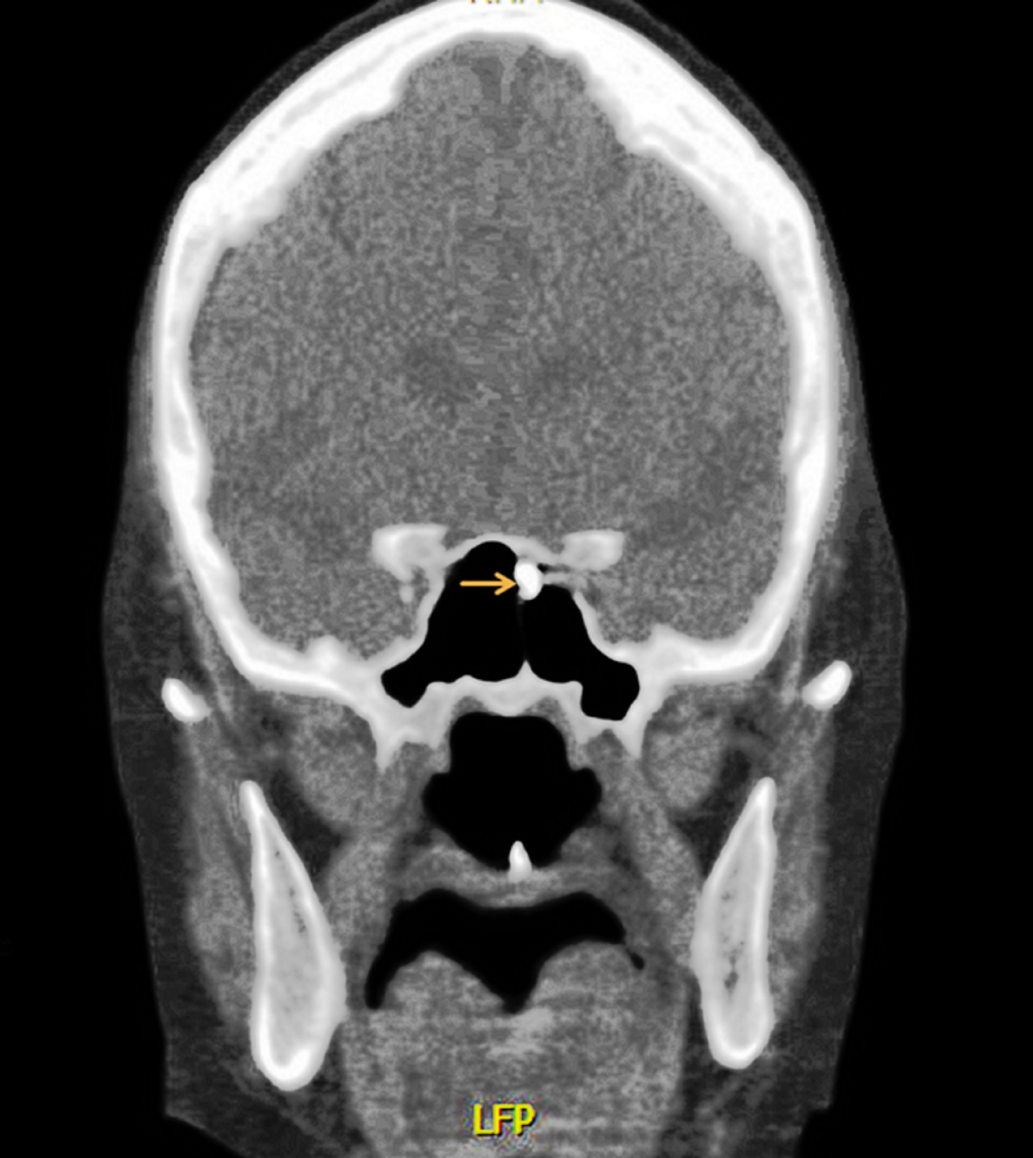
Computed tomography coronal view through the sphenoid sinus showing an oval, well-circumscribed, hyperdense opacity (arrow) in the superomedial aspect of left sphenoid sinus, consistent with the dropped magnet.
